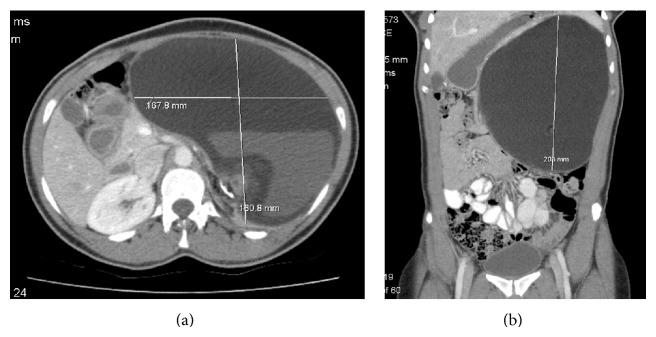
CT images of a giant left retroperitoneal teratoma: (a) cross-sectional view and (b) coronal view.
